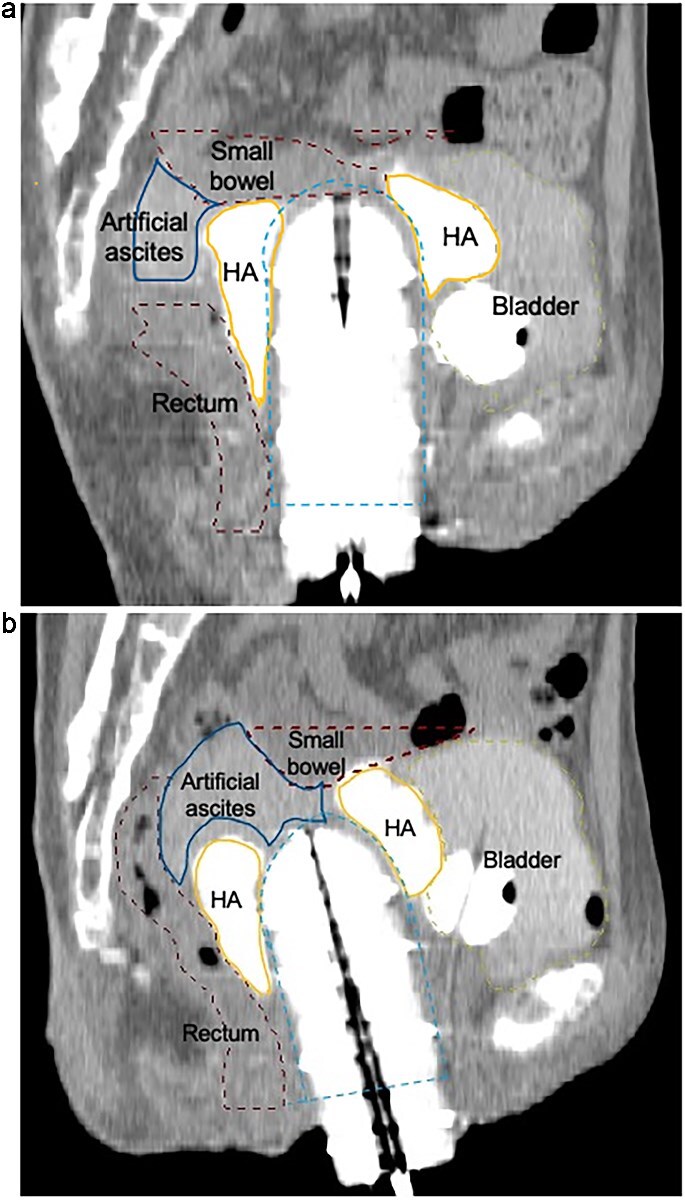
(a) Sagittal CT image acquired during the initial HDR brachytherapy session. Artificial ascites (blue contour, lateral) was instilled intraperitoneally to separate the small bowel (red dashed contour, superior) from the high-dose region, and hyaluronic acid (HA) gel (orange contour, bilateral anterior) was injected into the vesicovaginal and rectovaginal spaces to displace the bladder and rectum. Despite these efforts, the small bowel remained in close proximity to the target volume. (b) Sagittal CT image acquired during the fourth fraction of the initial HDR brachytherapy. To reduce radiation exposure to adjacent organs, HA gel (orange contour, bilateral anterior) was injected into both the vesicovaginal and rectovaginal spaces, and artificial ascites (blue contour, lateral) was instilled to displace the small bowel (red dashed contour, superior). In this fraction, a greater cranial shift of the small bowel was observed compared to earlier fractions, indicating improved displacement efficacy. The bladder and rectum are delineated by yellow (anterior) and dark red (posterior) dashed contours, respectively. Line styles and anatomical positions are provided alongside color for accessibility.
